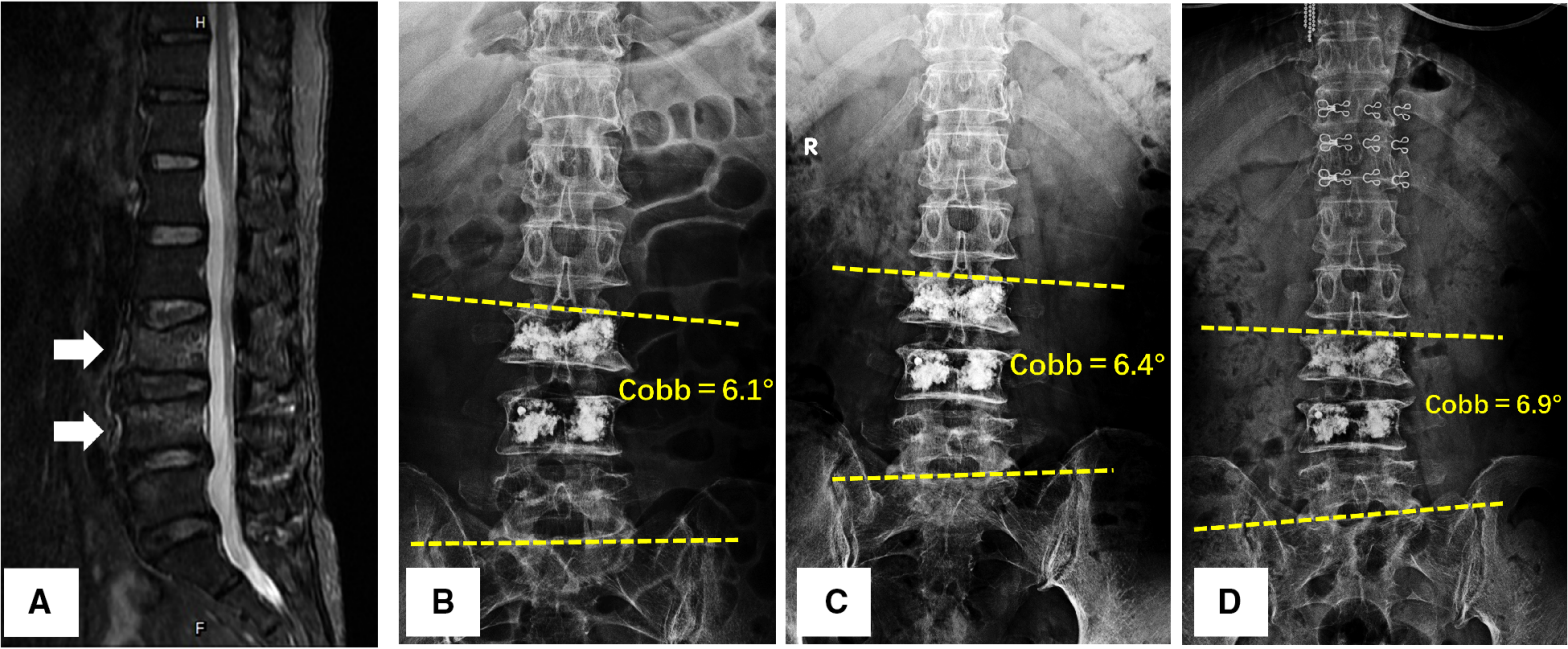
A 64-year-old female was diagnosed with L3 and L4 vertebral compressed fractures treated with bilateral PVP. (A) Preoperative T2-weighted MRI scan showed a higher-intensity signal within the fractured vertebral bodies; (B–D) 24 h after surgery, 6-month follow-up, and 48-month follow-up anteroposterior *x*-rays of lumbar spine, coronal segmental cobb angle varied little over time.
